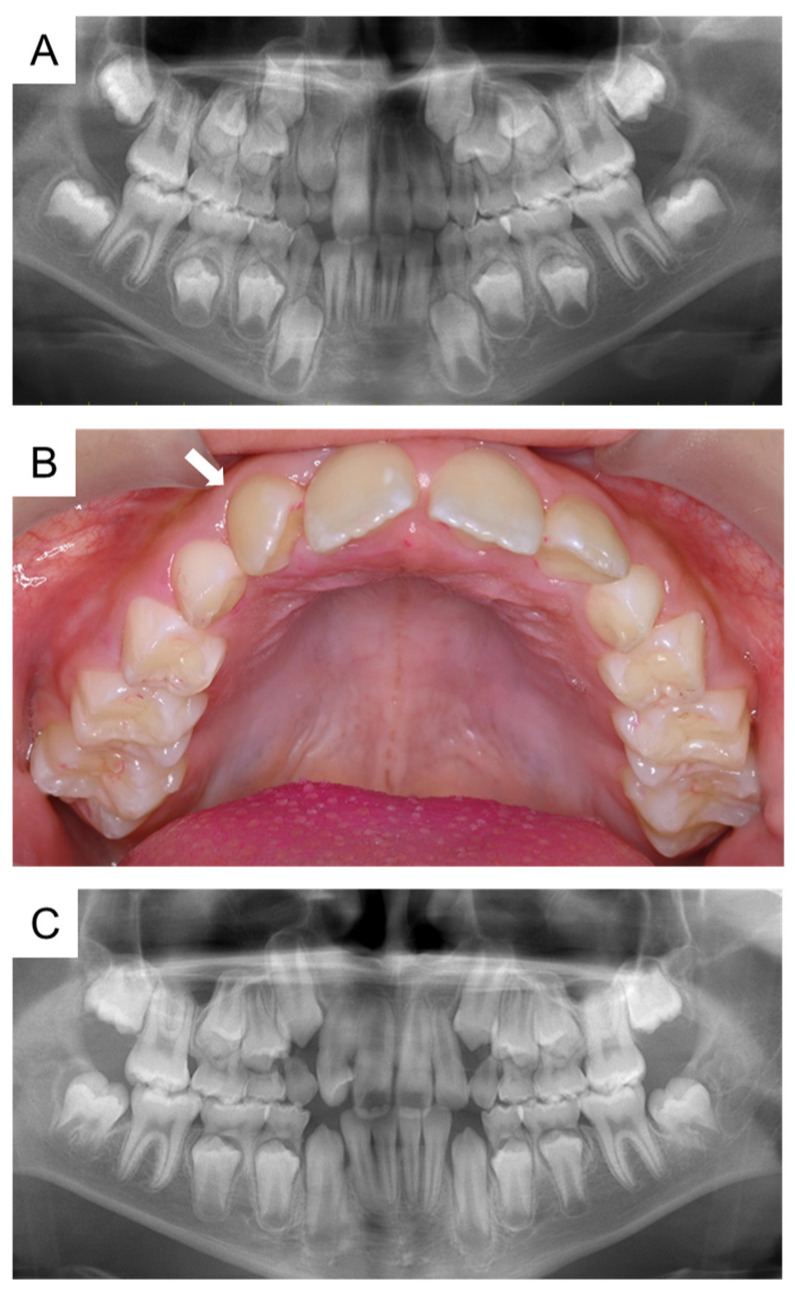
Panoramic radiographs and intraoral examination radiographs before and after the eruption of the maxillary permanent incisors. (A) Panoramic radiograph at the age of 8 years and 10 months. (B) Intraoral photograph at the age of 10 years and 6 months. Arrows indicate the maxillary right lateral incisor. (C) Panoramic radiograph at the age of 10 years and 10 months.
